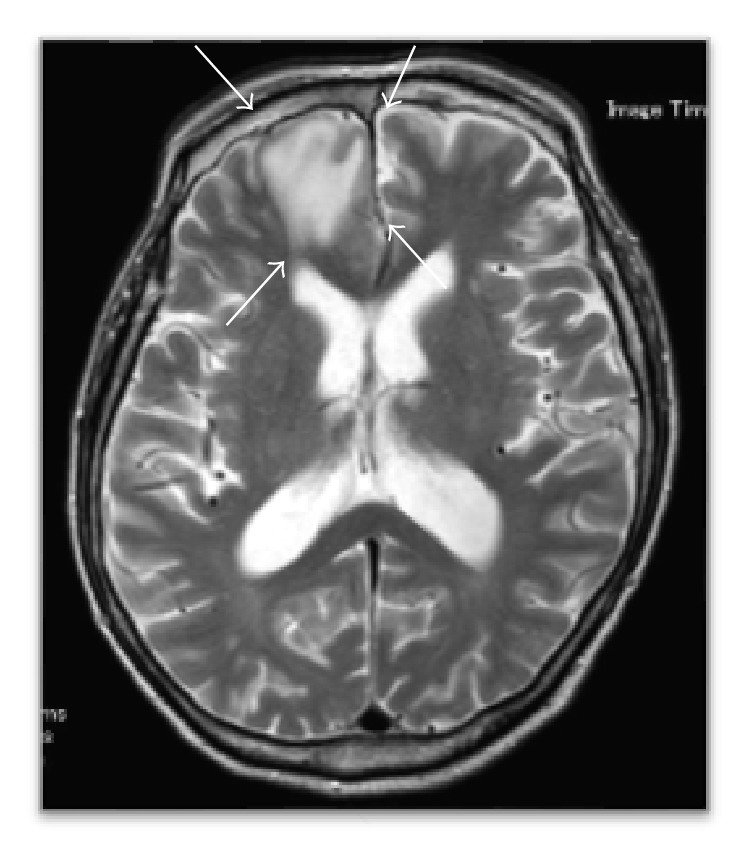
MR images (T2WI) showing hypointensity lesion in the right frontal lobe.
